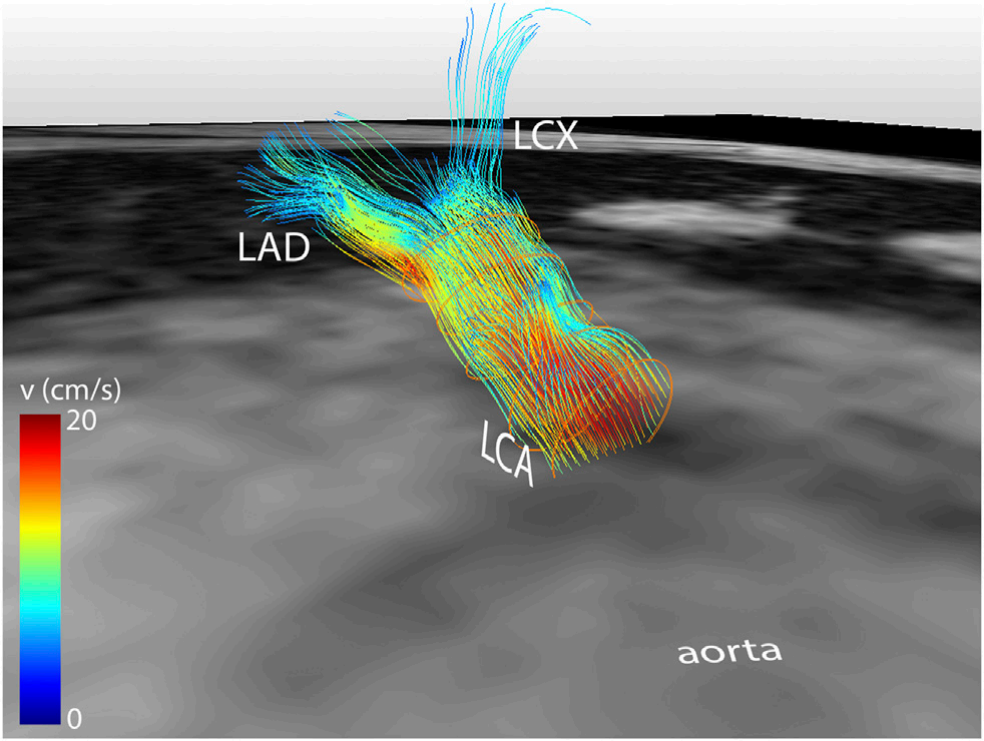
Streamline reconstruction of 4D flow MRI-derived velocities in the LCA for a mid-diastolic time frame. Streamlines initiate from five contours placed in the LCA and split into LAD and LCX. Velocity color-coding shows that the measured velocities in the LAD and LCX are lower than in the LCA.
